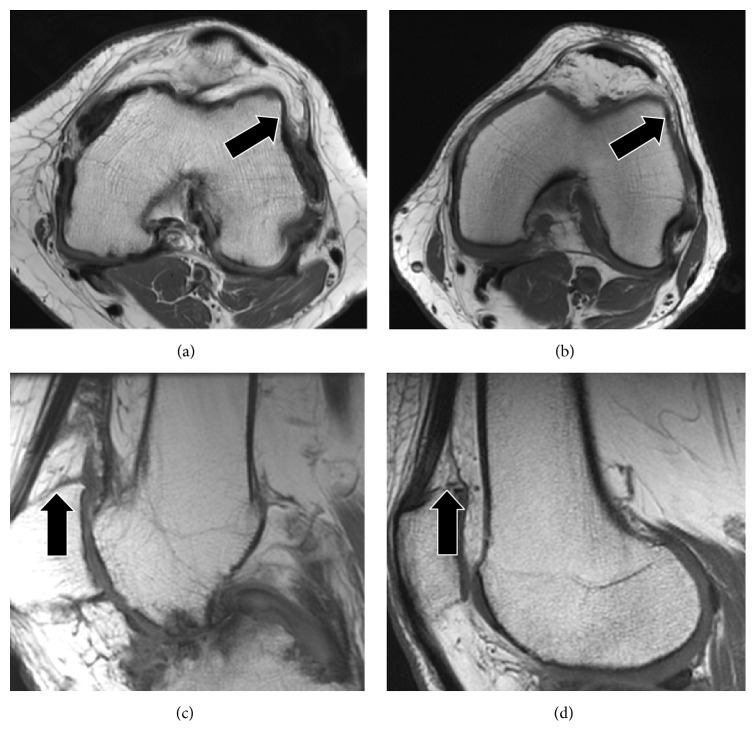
T1-weighted MR images showing different infrapatellar fat pad (IPFP) and suprapatellar fat pad (SPFP) appearances. (a) 48-year-old man with severe hemophilia B demonstrates an enlarged IPFP with prominent extension into the distended lateral patellofemoral recess (arrow). (b) 70-year-old man with mild hemophilia A shows a more regular appearance of the IPFP with only mild extension into the lateral patellofemoral recess (arrow). (c) 38-year-old man with severe hemophilia A demonstrates an enlarged and irregular SPFP (arrow). (d) 67-year-old man with mild hemophilia A shows a more regular appearance of the SPFP (arrow).
